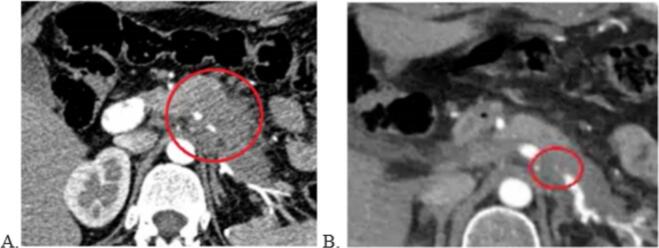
Contrast-enhanced computed tomography (CT) of the abdominal cavity. A) CT of the abdominal organs before chemotherapy, 26.04.2022. B) CT of the abdominal organs after completion of chemotherapy, 21.02.2023.
